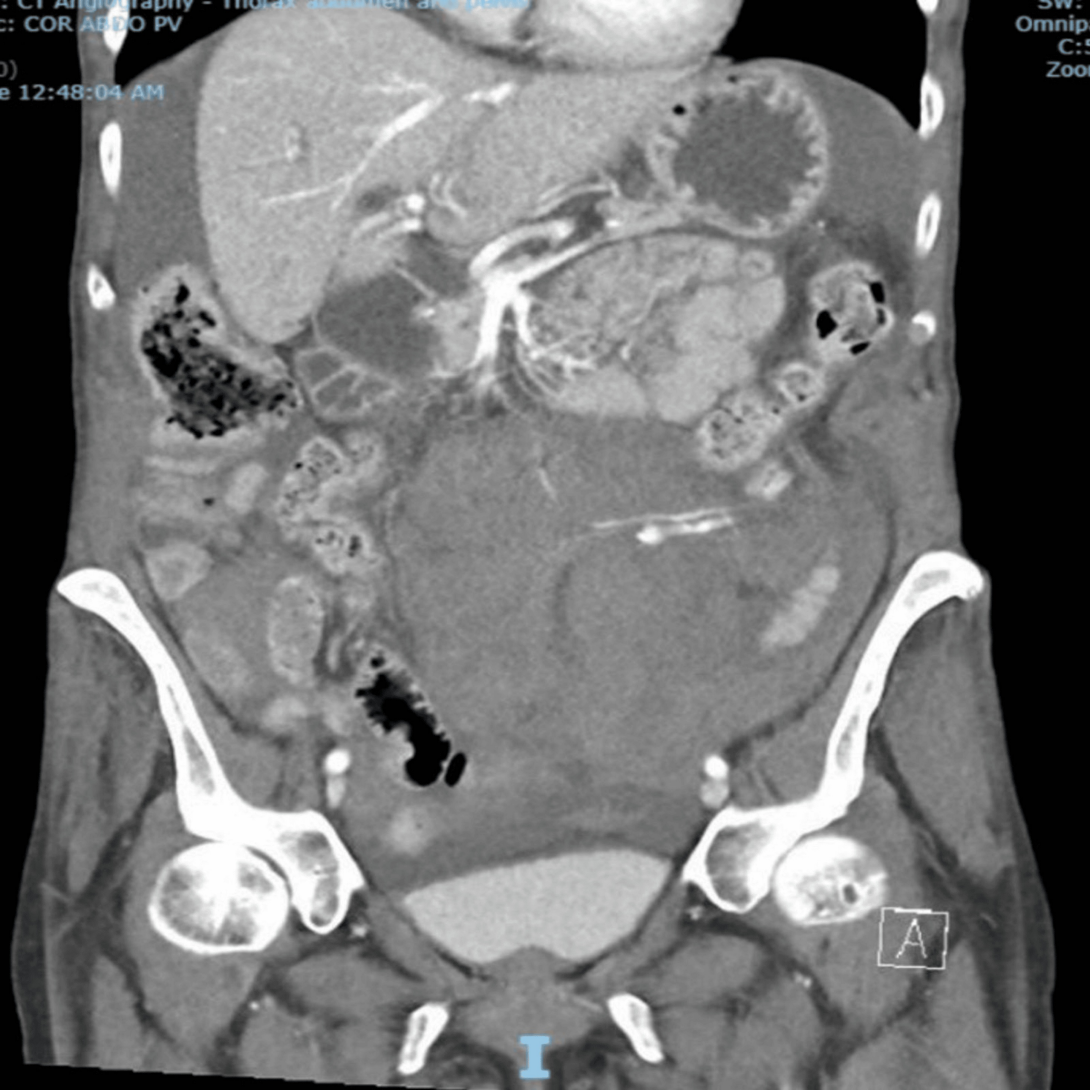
CT scan performed ~18 hours later showing large volume hemoperitoneum and hematoma with active bleeding CT: computed tomography
